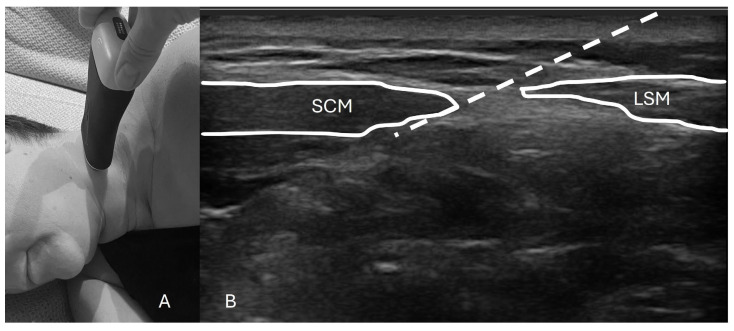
Probe placement and ultrasound image with landmarks. (A) The patient is positioned in the lateral decubitus position with the ultrasound probe placed approximately at the middle third of the sternocleidomastoid muscle (SCM). (B) An axial ultrasound scan of the cervical area shows the SCM and levator scapulae muscle (LSM). The white striped line indicates the needle trajectory, with the final dot marking the anatomical target for the injection.
